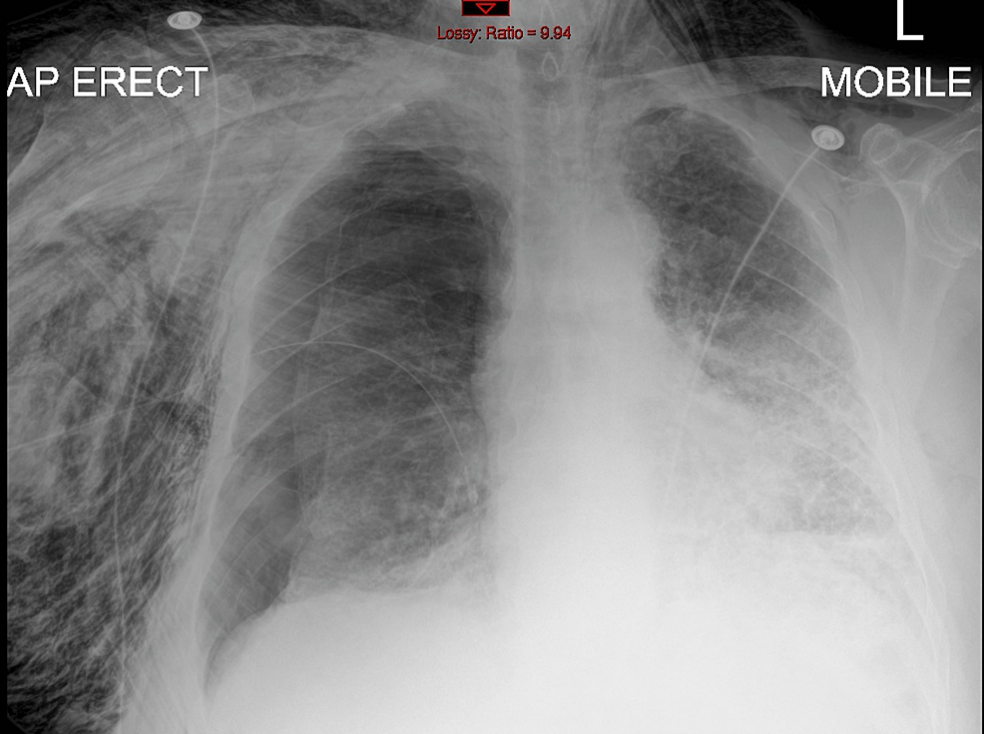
Decrease in the size of the right-sided pneumothorax, with concomitant increase in subcutaneous emphysema. Anterior-posterior (AP), chest X-ray with chest drain on the right side.
